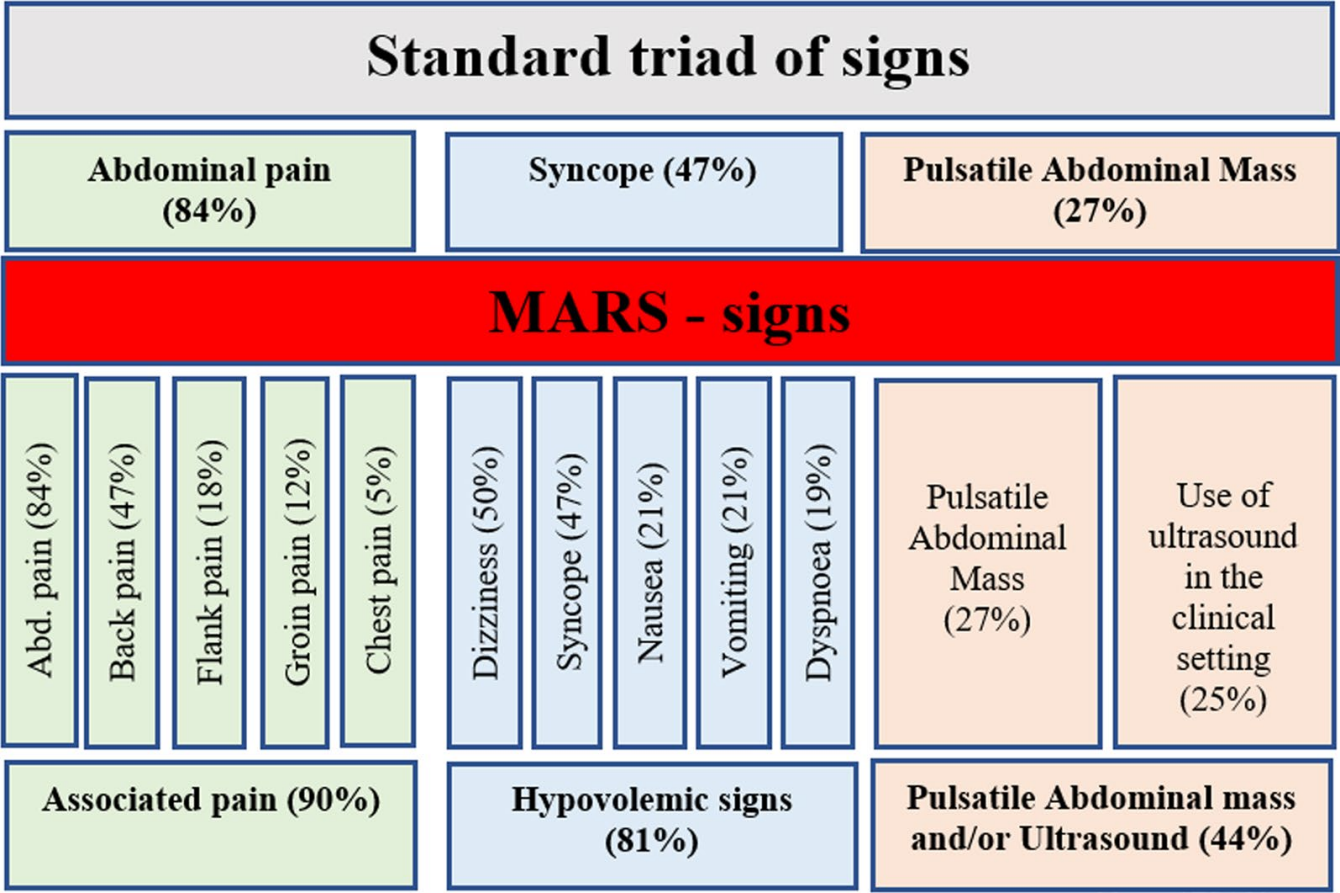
Summary and prevalence of symptoms for STS and MARS. Standard triad consist of abdominal pain, syncope and pulsatile abdominal mass. MARS includes all registries of any localization pain, expression of hypovolemic signs and addition of sonography or examination of pulsatile abdominal mass
